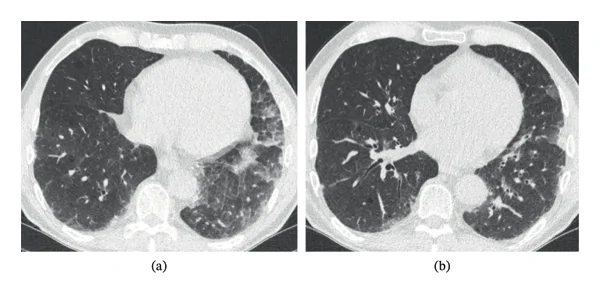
Unenhanced transverse thin‐section (0.625 mm) CT at lung windows with low‐dose settings. (a), (b) Subpleural ground glass, consolidation, and interlobular septal thickening with a basal predominance.
